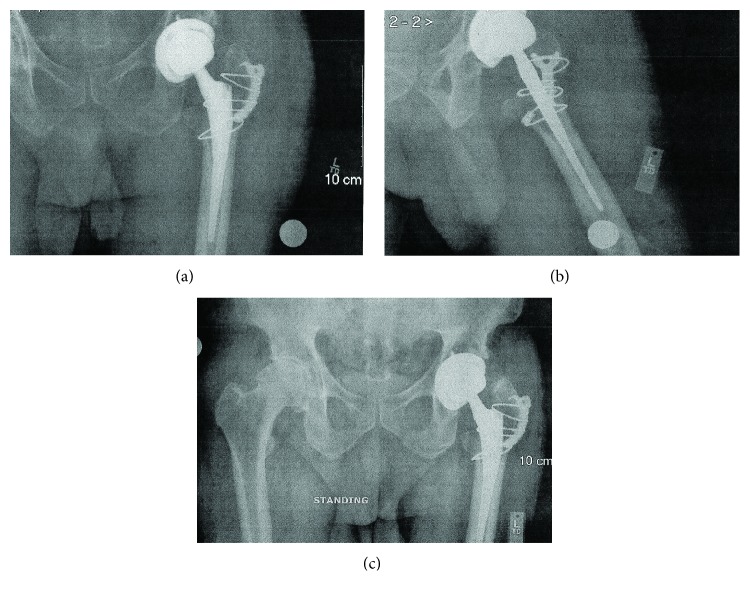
AP and lateral hip and standing pelvic X-ray at a six-week postoperative visit that demonstrate satisfactory positioning of the implants and trochanteric cable plate.
